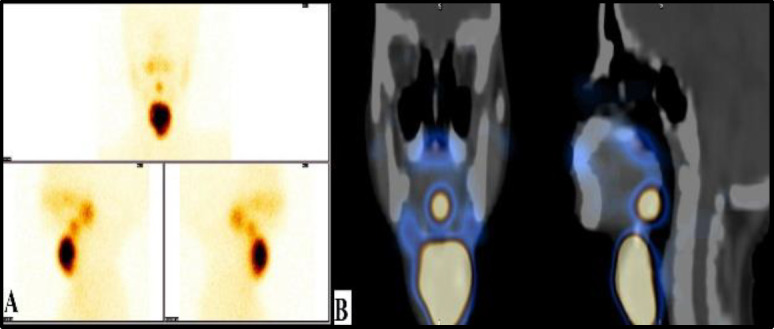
A. Tc-99m thyroid scintigraphy of a patient shows double ectopic thyroid, involving the sublingual and infrahyoid regions. B. SPECT-CT (coronal section and Sagittal section) of the neck region confirms the anatomical location of the ectopic thyroid tissue
